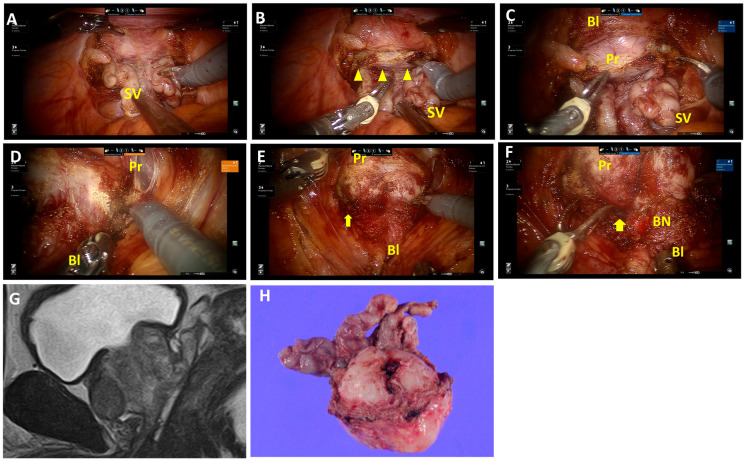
Surgical techniques of the retrotrigonal layer dissection from the posterior approach and bladder neck preservation in patients with an enlarged prostate. (A) Isolation of the seminal vesicle and dissection of deferens vasa in a posterior approach. (B) The retrotrigonal layer, pinkish longitudinal tissue, is transected (triangles). (C) The surface of the prostatic pseudocapsule can easily be identified after transection. This layer can be developed anteriorly. (D) Right lateral approach in bladder neck dissection. (E) Detrusor muscles are detached from the prostate. The posterior space is visible laterally (arrows). (F) The bladder neck is preserved (arrows). (G,H) MRI sagittal view and macroscopic findings of the prostate and seminal vesicle. Enlargement of the prostate gland is visible and the surface of the prostatic pseudocapsule at the protrusion site is exposed (H). SV, seminal vesicles; Bl, bladder; Pr, prostate; BN, bladder neck.
